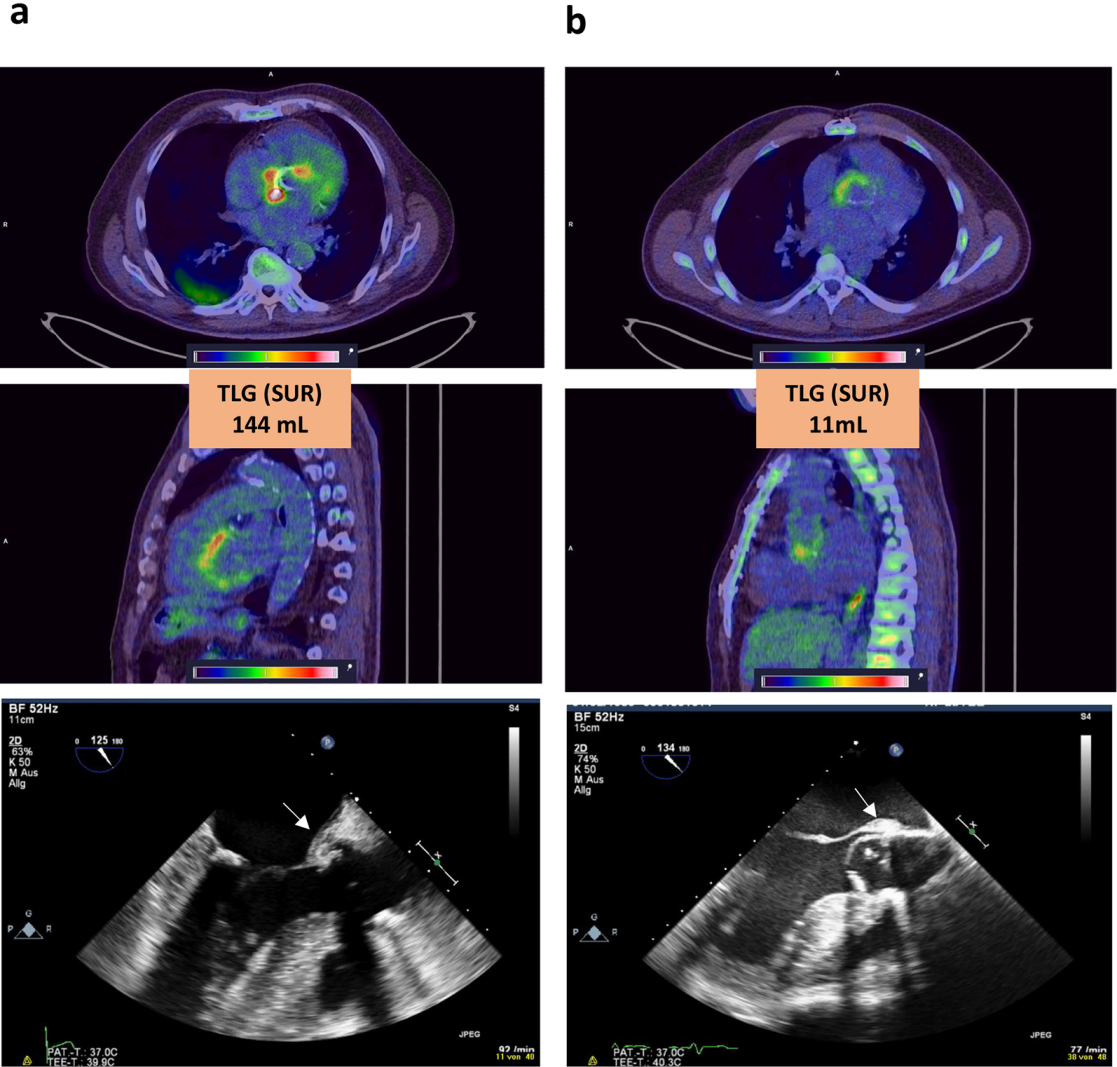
^18^F-FDG PET/CT scans of two patients with surgically confirmed IE. ^18^F-FDG PET/CT scans in the *upper* and *middle panel* demonstrating increased TLG (SUR) in a patient with abscess formation (a) as compared to a patient without surgically confirmed abscess formation (b). The echocardiographic images are shown in the *lower panel*. The arrow in a points at aortic root abscess formation. The arrow in b points at a suspicious structure in the area of the aortic root in a patient that had David surgery four years ago that turned out to be no abscess upon surgery. Color scale of PET windowed with SUV 0 to 8. *SUR* standard uptake ratio, *TLG* total lesion glycolysis
